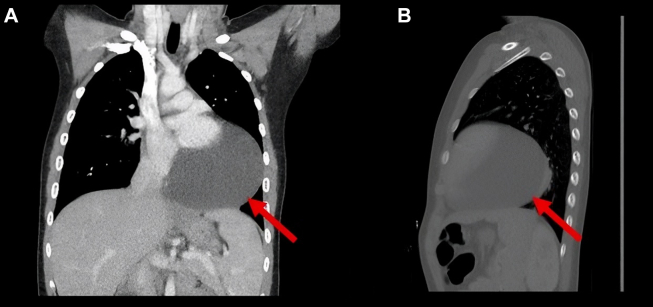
CT Chest With Contrast Computed tomography chest with contrast with coronal plane (A) and sagittal plane (B), revealing a large 8 × 7.8 × 8.9 cm pericardial cyst with mass effect on the heart (red arrows) and adjacent compressive atelectasis of the left lower lobe.
